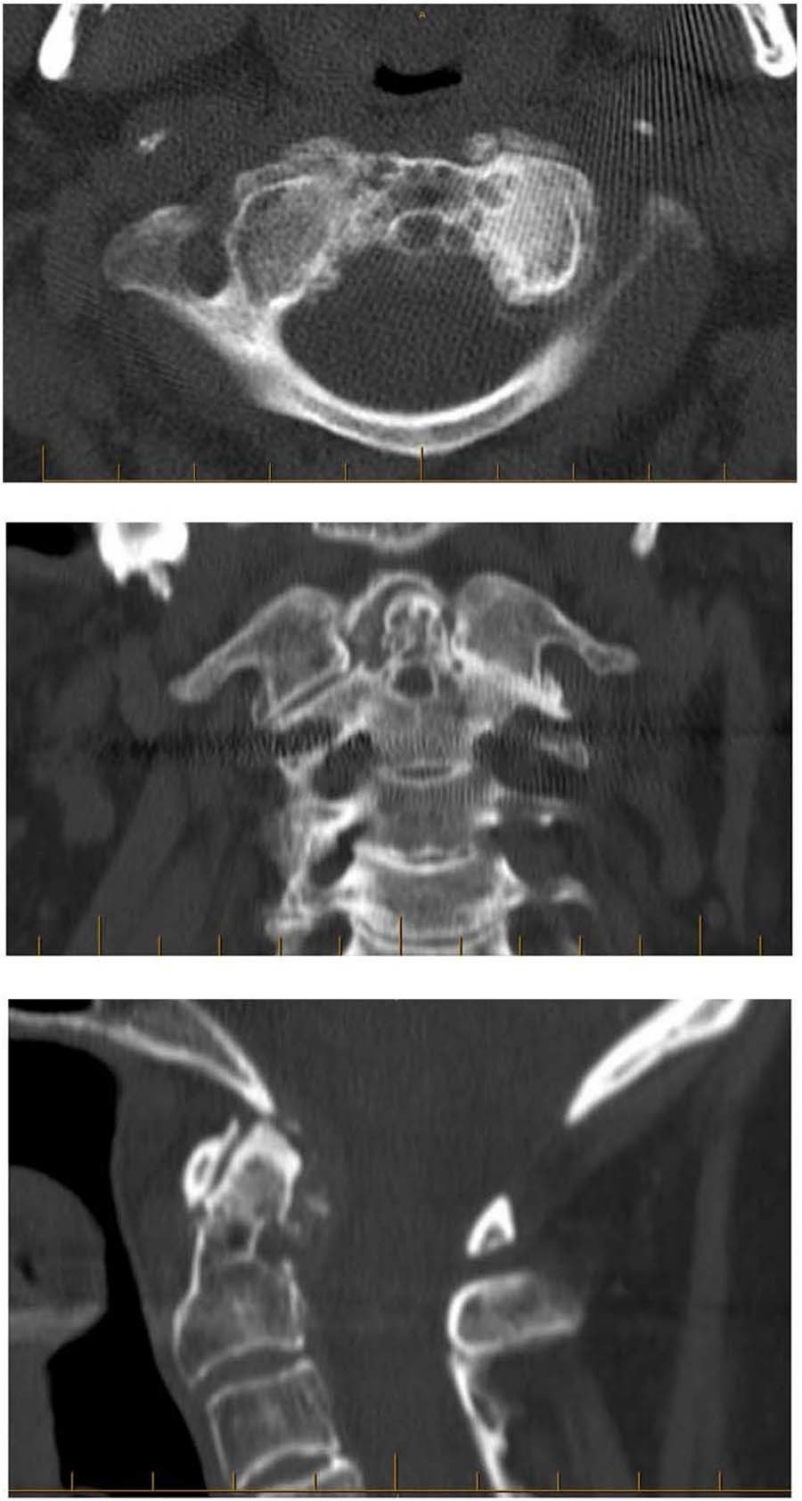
Preoperative images (CT scans)
